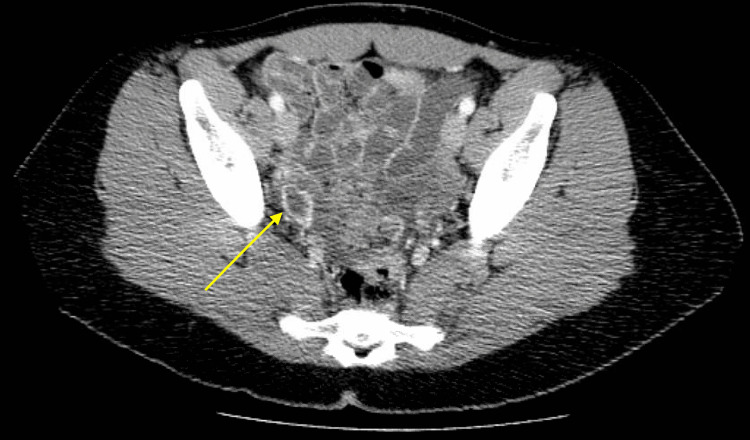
Computed tomography scan of the abdomen and pelvis, which revealed a crenulated right ovarian lesion (indicated by the yellow arrow)
